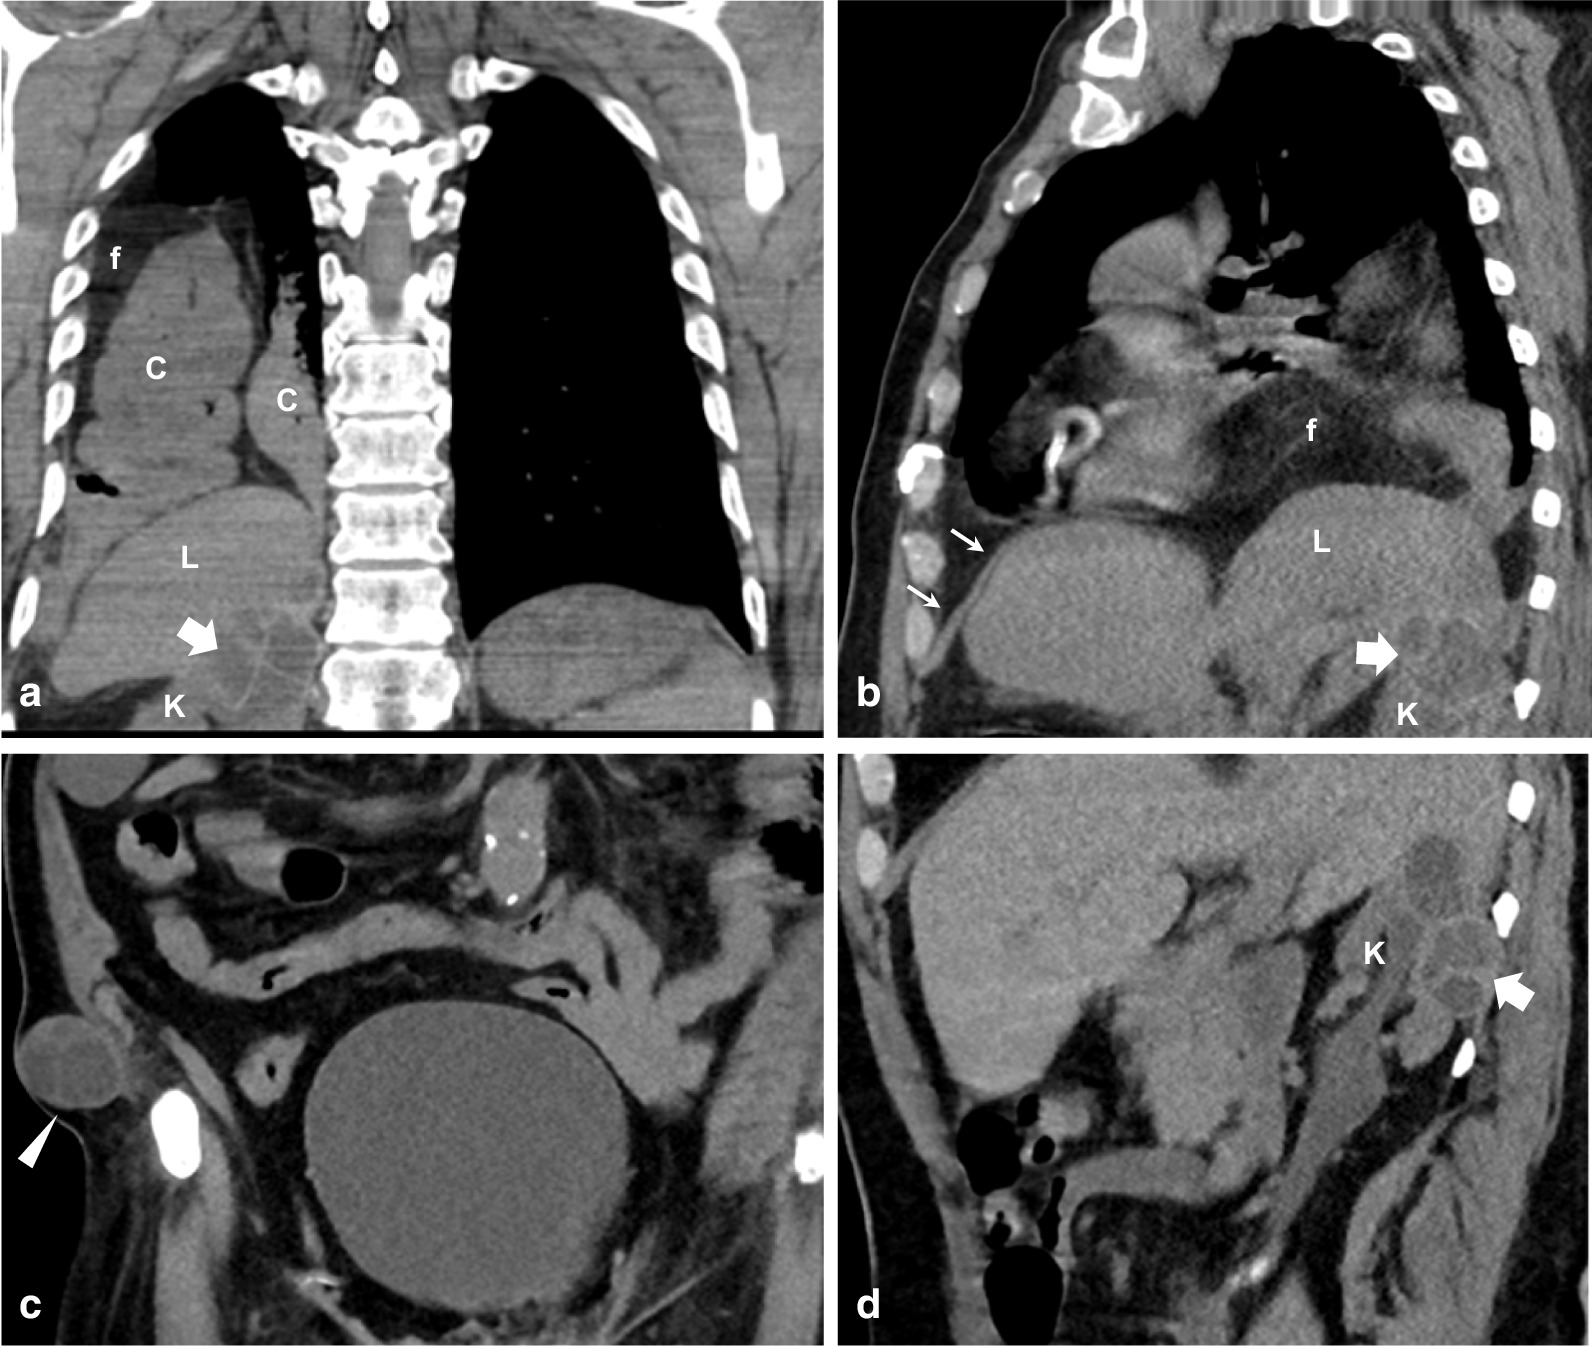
Unenhanced computed tomography scan of the chest (a coronal and b sagittal) and of the abdomen (c coronal and d sagittal), done on different dates, showing colonic loops (C in a) and accompanying fat (F in a, b) within the right hemithorax. The anterior portion of the diaphragm is present (thin arrows in b) over the liver (L), while the posterior portion is absent. Imaged portions of the upper abdomen show an exophytic multilocular/complex cystic lesion (thick arrows in a, b, and d), arising from the upper pole of the kidney (K) and abutting the liver (L). A similar lesion is seen in the subcutaneous tissue of the lower abdomen (arrowhead in c)
